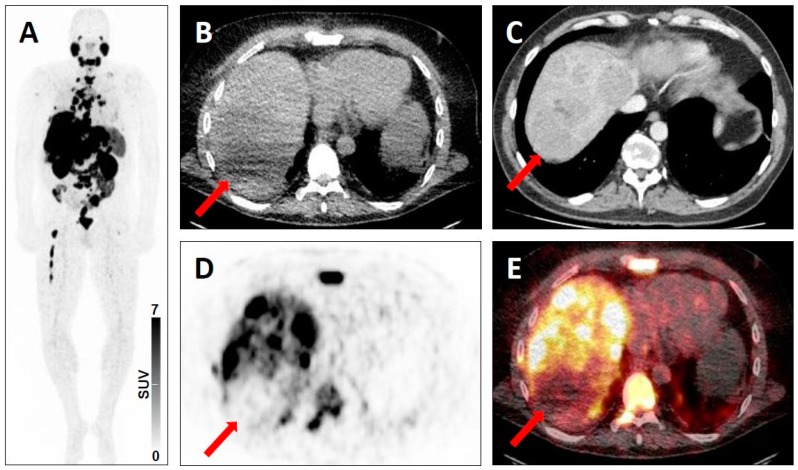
Lesion suggestive of malignancy on anatomic imaging but lacking uptake on PSMA-targeted positron emission tomography (PET)/computed tomography (CT). ^68^Ga-PSMA-11 PET/CT for restaging in a 52-year-old man with a history of a prostate cancer. Whole-body maximum intensity projection image demonstrating intense radiotracer uptake in metastases in the liver, lymph nodes and bone (A). Axial low-dose CT (B), contrast-enhanced CT (C), PET (D), and fused PET/CT (E) showing a hepatic lesion suggestive of malignancy on anatomic imaging, but lacking uptake (red arrows), consistent with a PSMA-RADS-3D lesion. In addition, there are numerous hepatic lesions with intense tracer uptake, consistent with a classification as PSMA-RADS-5 lesions.
